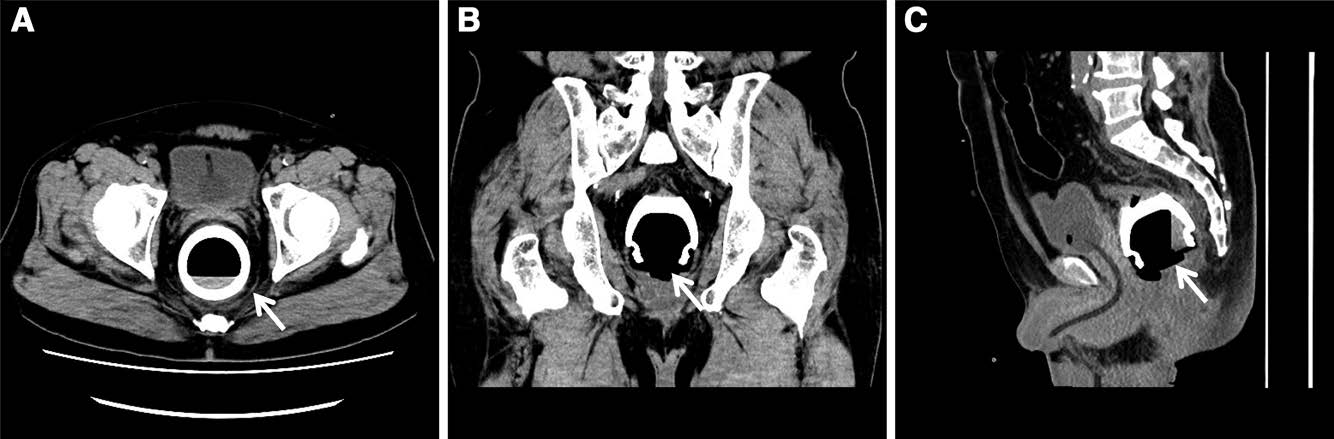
CT imaging shows a cup-shaped foreign body stuck in the rectal lumen (white arrow). (A) Axial view, (B) coronal view, (C) sagittal view. CT = computed tomography.
